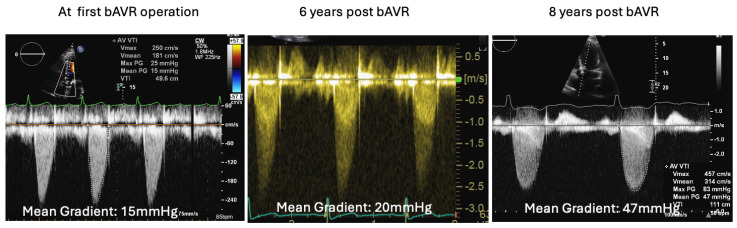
Series of transthoracic echocardiographies in valve clinic—CW measurements of transvalvular gradient across AV bioprosthesis since its implantation. Mean transvalvular gradient showed progressive increase suggestive of severe degenerative AS.
